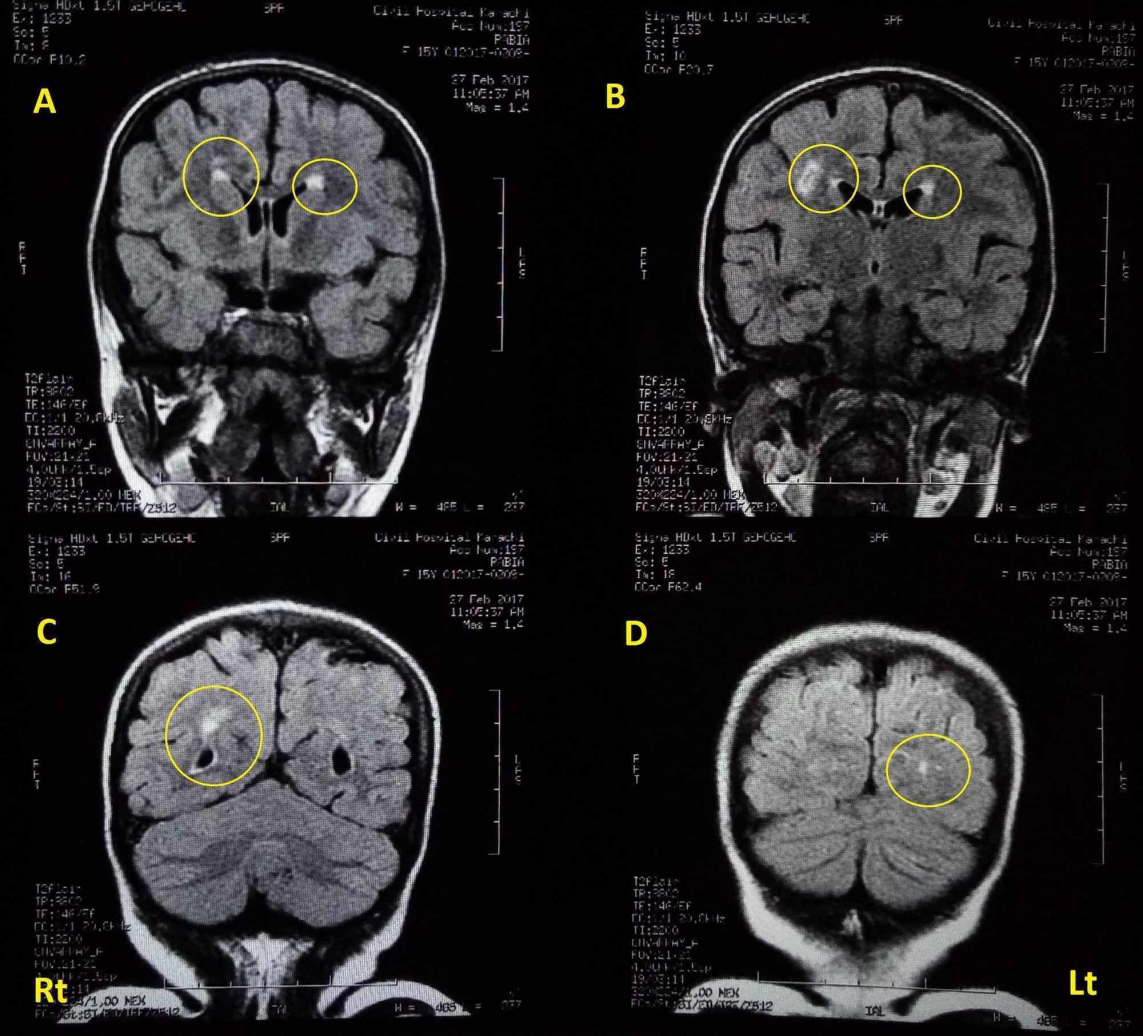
Case one: MRI Brain showing changes consistent with occlusive arteriopathy, with attenuation of bilateral middle and posterior cerebral circulation Areas of involvement, encircled in the image, can be prominently seen in the MRI FLAIR sequence. Yellow circles indicate areas of old paraventricular ischemia/infarction MRI: magnetic resonance imaging; FLAIR: fluid-attenuated inversion recovery; Rt: anatomical right side of the brain; Lt: anatomical left side of the brain
